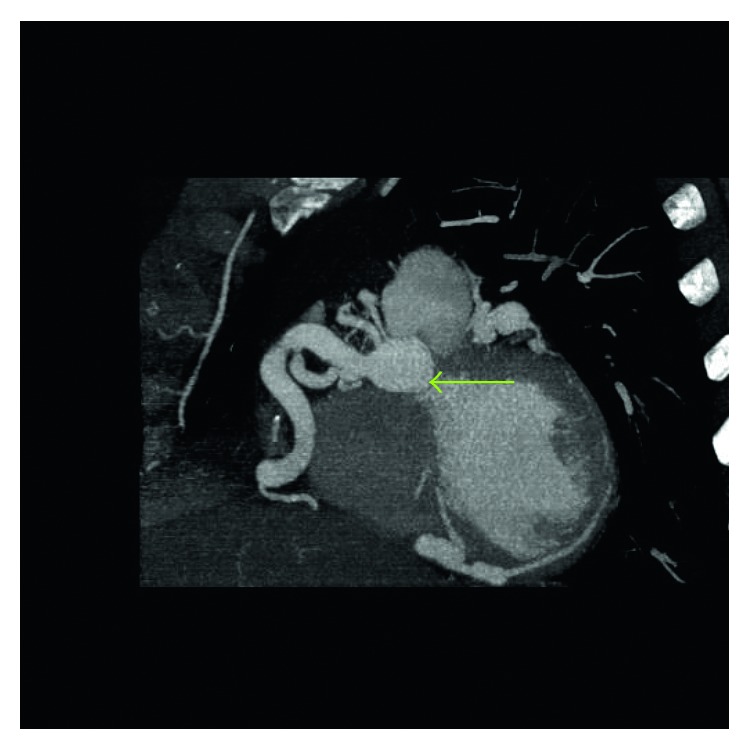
Sagittal view of multidetector cardiac CT angiogram shows an ectatic and dilated right coronary artery (RCA) (green arrow).
